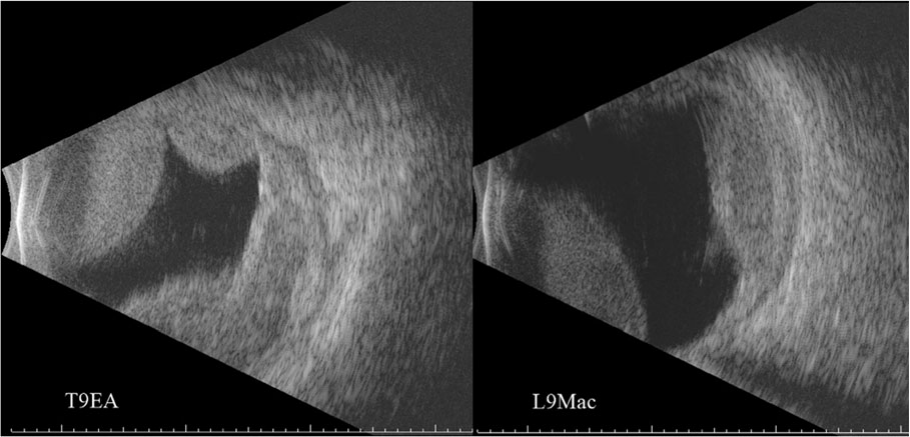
Ultrasound B-Scan of the right eye showing extensive suprachoroidal haemorrhage involving the center of the macula
